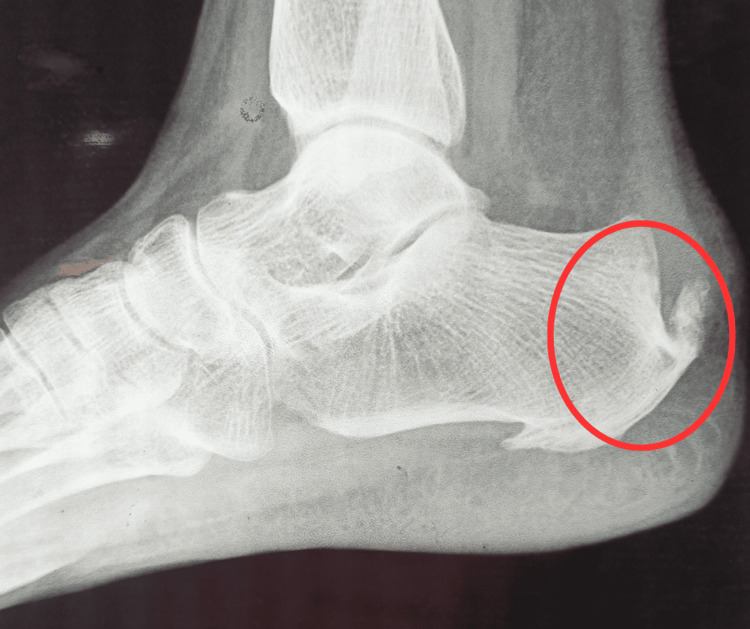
Radiographic representation of the lateral view of the right-side ankle joint, which shows calcaneal spur.
